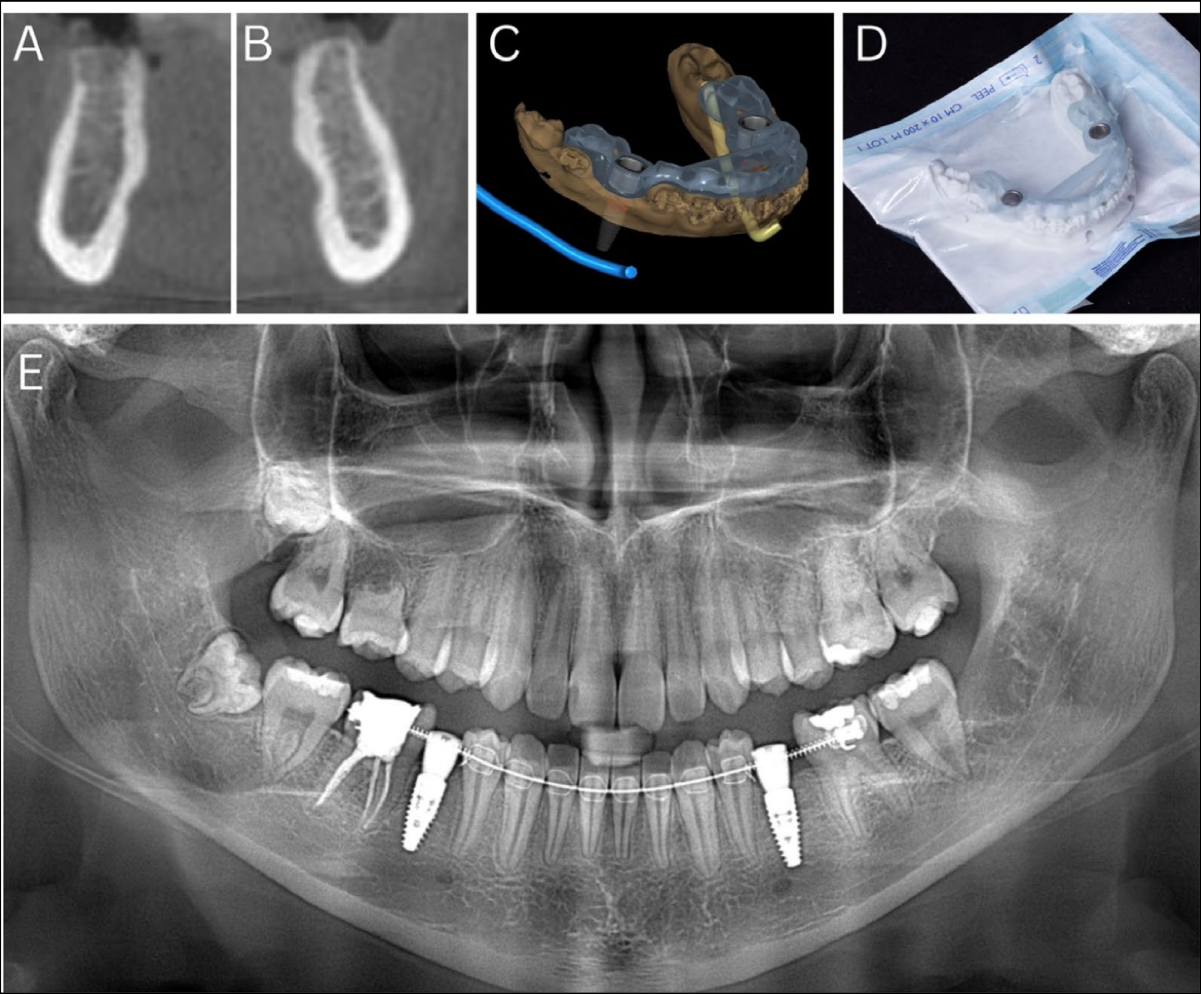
Digital planning and implant placement in agenesis sites using three-dimensionally printed surgical guide. A, B Cone-beam computed tomography cross-sectional images of mandibular sites 45 (A) and 35 (B), showing ridge dimensions following orthodontic space opening in areas of congenital agenesis of the second premolars. C Digital planning of implant positioning and surgical guide design based on cone-beam computed tomography and intraoral scan data. D Sterilized three-dimensionally printed surgical guide fabricated for use during implant placement. E Postoperative panoramic radiograph showing placement of Straumann bone level tapered implants (diameter 4.1 mm, length 10 mm) in sites 35 and 45 via guided flap surgery
